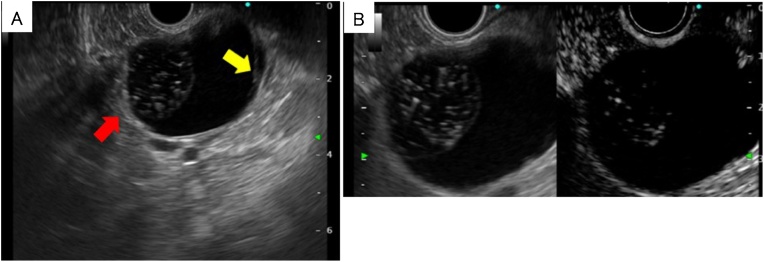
Endoscopic ultrasonography (EUS) revealing a cystic lesion in the region of the pancreatic tail and suggestive of internal septa (red arrow) and intramural nodular structure showing mixed low- and high-echoic areas (yellow arrow) (A). Enhanced EUS detected no enhancement in the whole lesion (B).
